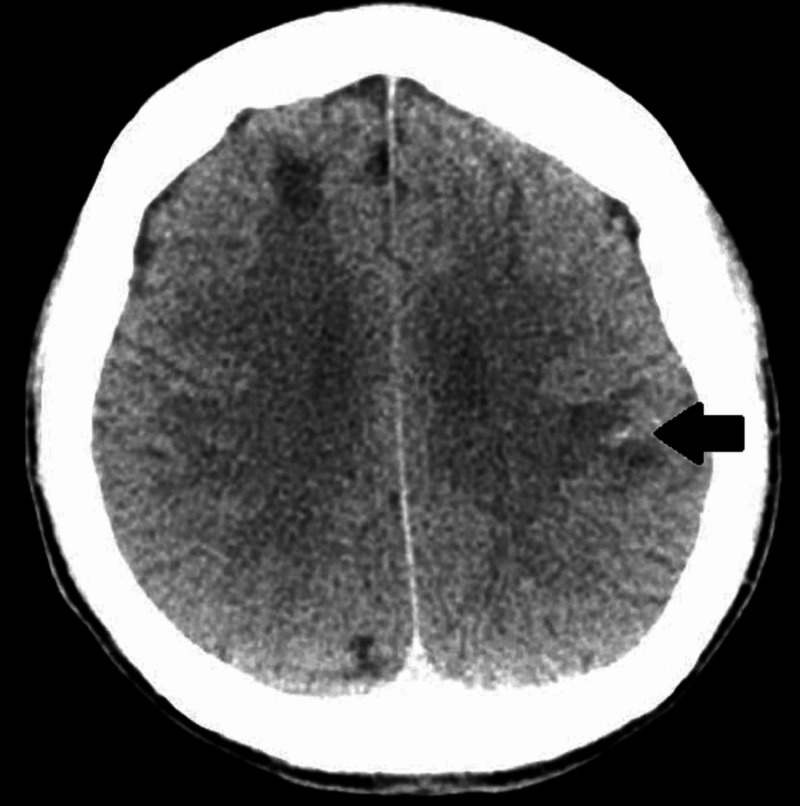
Computed tomography of head showing punctate hypointensity in the left temporoparietal region (black arrow)
